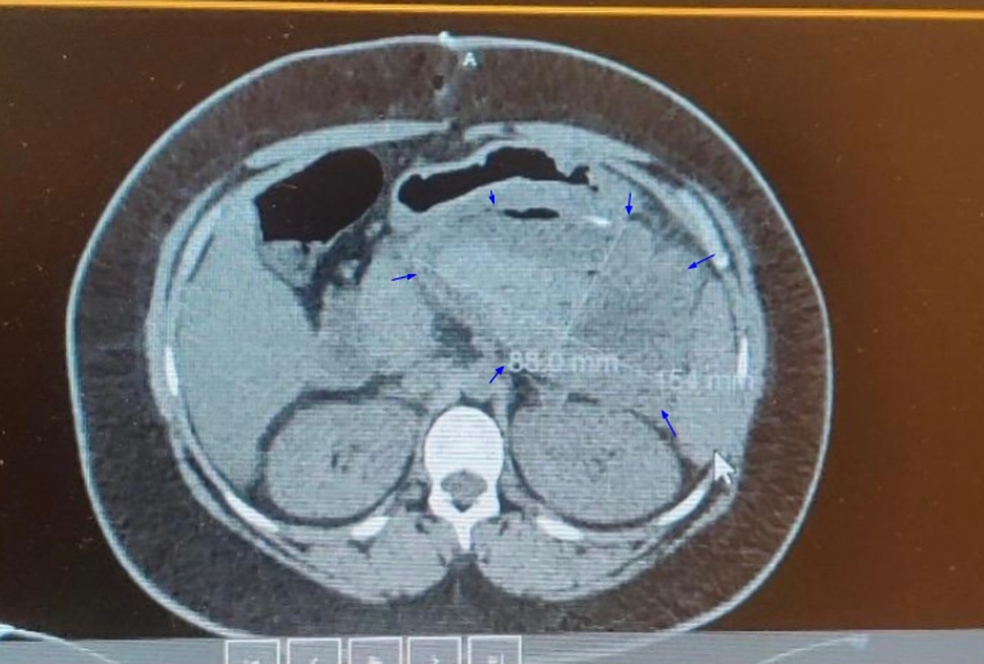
Non-contrast CT scan of the abdomen on postoperative day 2. Blue arrows point toward the boundaries of the pancreatic cyst, and the cyst is filled with inflammatory debris, which appears as hypodense on the CT scan.
